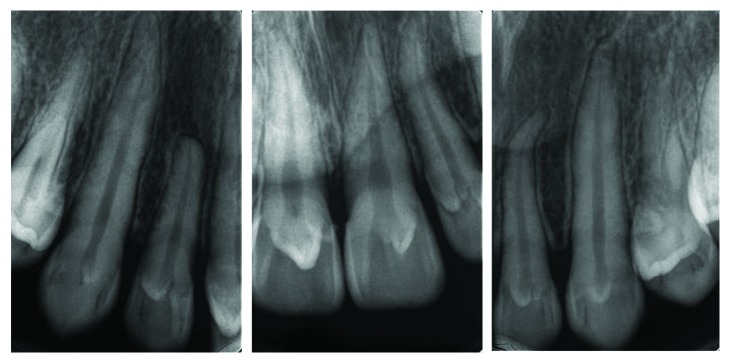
Case 3: periapical radiograph shows radiographic appearance of talon cusps.
